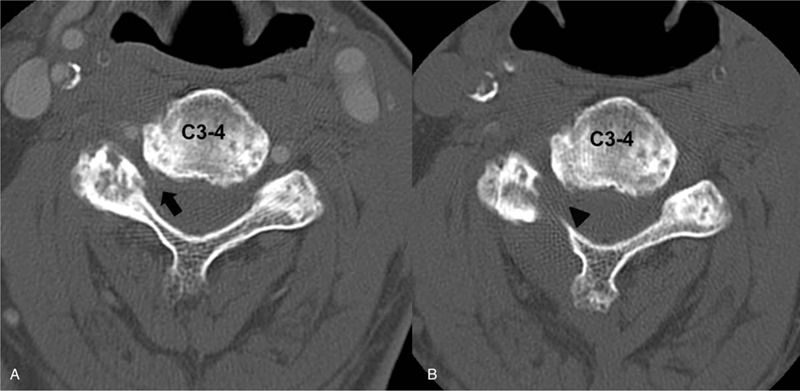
(A) Preoperative foraminal stenosis at C3–4 on the CT axial image. (B) Postoperative foraminal decompression at the C3–4 on the CT axial image. CT = computed tomography.
